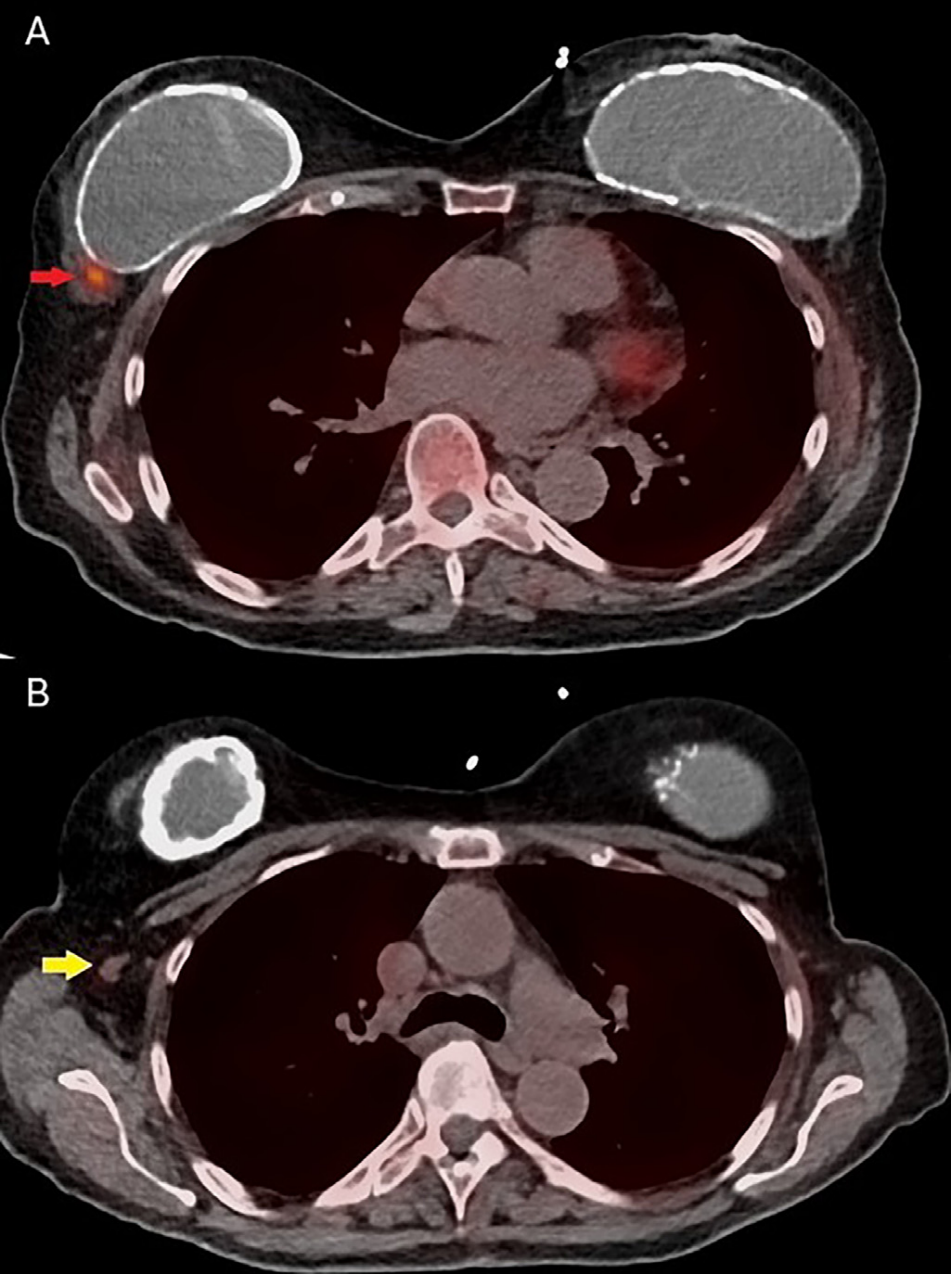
(A) Axial PET-CT image demonstrates uptake in the outer right breast along the implant capsule (red arrow). (B) There is mild uptake within a right axillary lymph node (yellow arrow).
